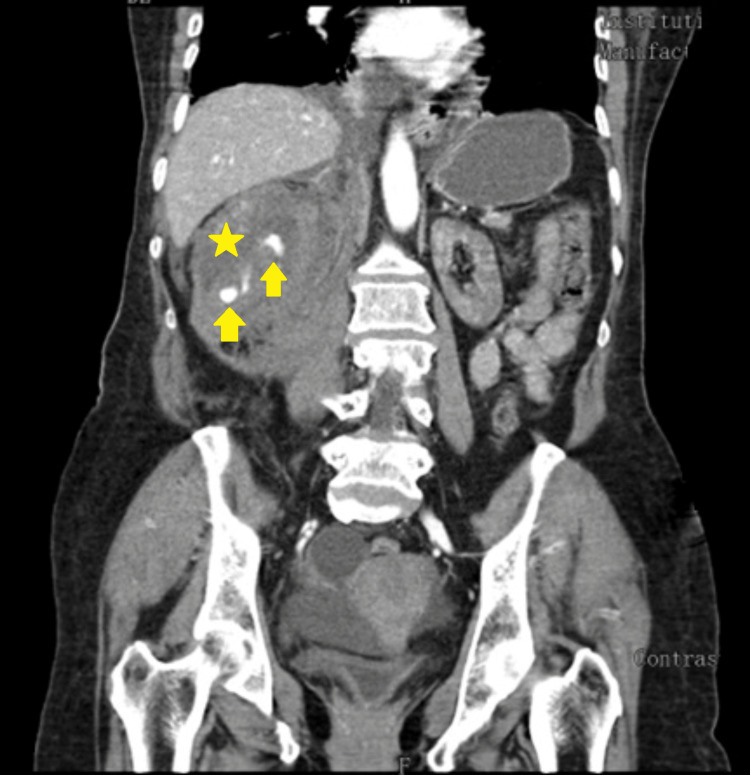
Right-sided renal and retroperitoneal hematoma - CT scan, coronal cut The hematoma (yellow star) occurred as a late complication of the kidney biopsy - four days after the procedure. The yellow arrows point toward the active extravasation of material, suggesting active hemorrhage.
